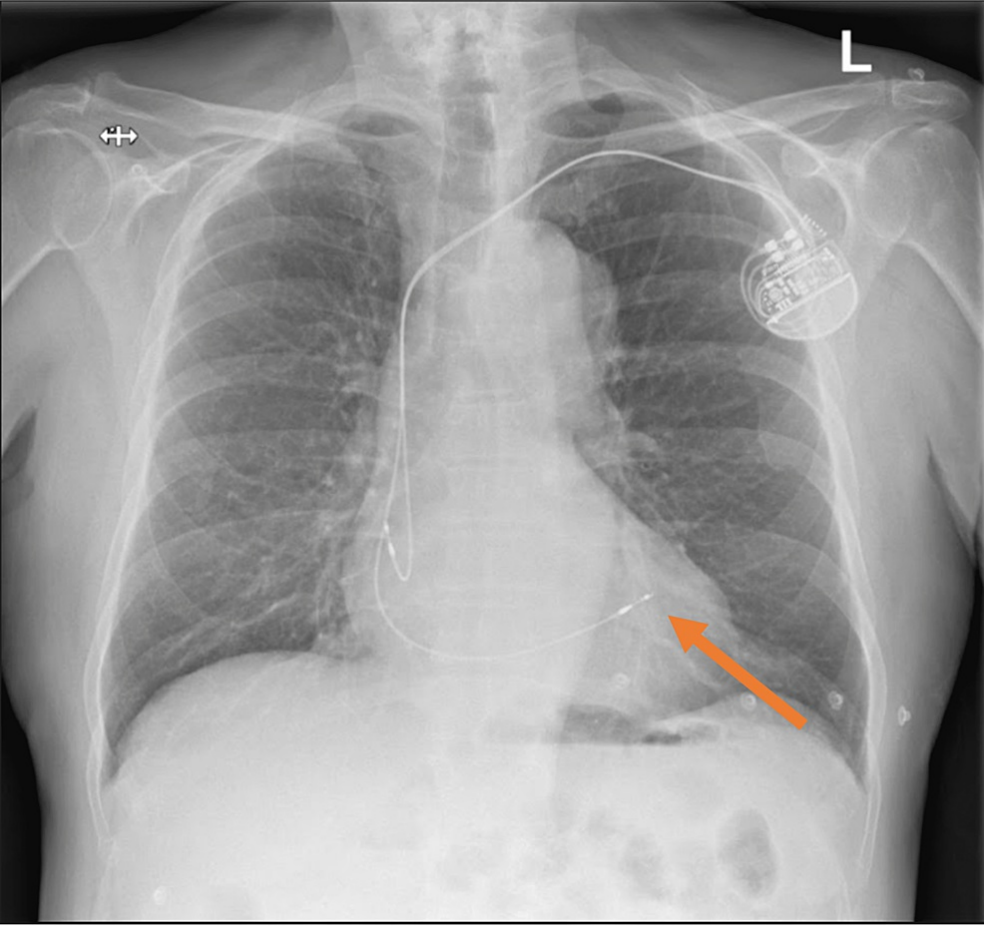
Chest radiograph on this admission. No evidence of pleural effusion/pneumothorax. The tip of the RV lead appears to be in a different position as compared to Figure 4. RV: right ventricular.
